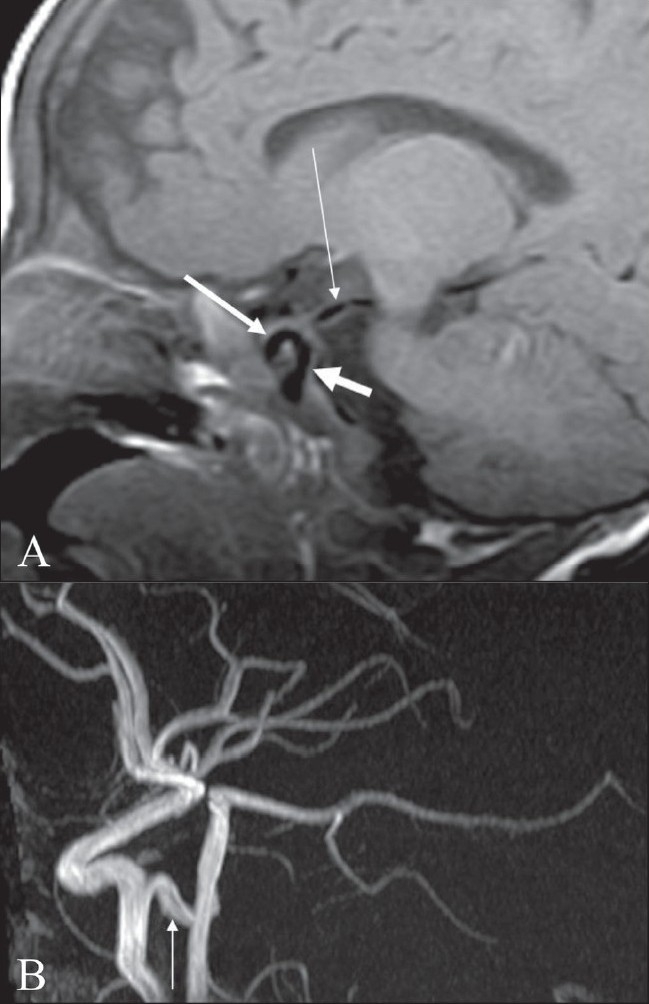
Tau sign. Sagittal T1-W image of the brain shows (A) flow voids of the internal carotid artery (ICA) in the precavernous segment (thick short arrow), in the cavernous segment (medium-sized arrow), and a persistent trigeminal artery (thin long arrow). Together, these flow voids form the Greek letter ‘τ’ (tau). Sagittal view of the MRI angiogram shows the persistent trigeminal artery (arrow) arising from the ICA and joining the basilar artery in its mid segment
